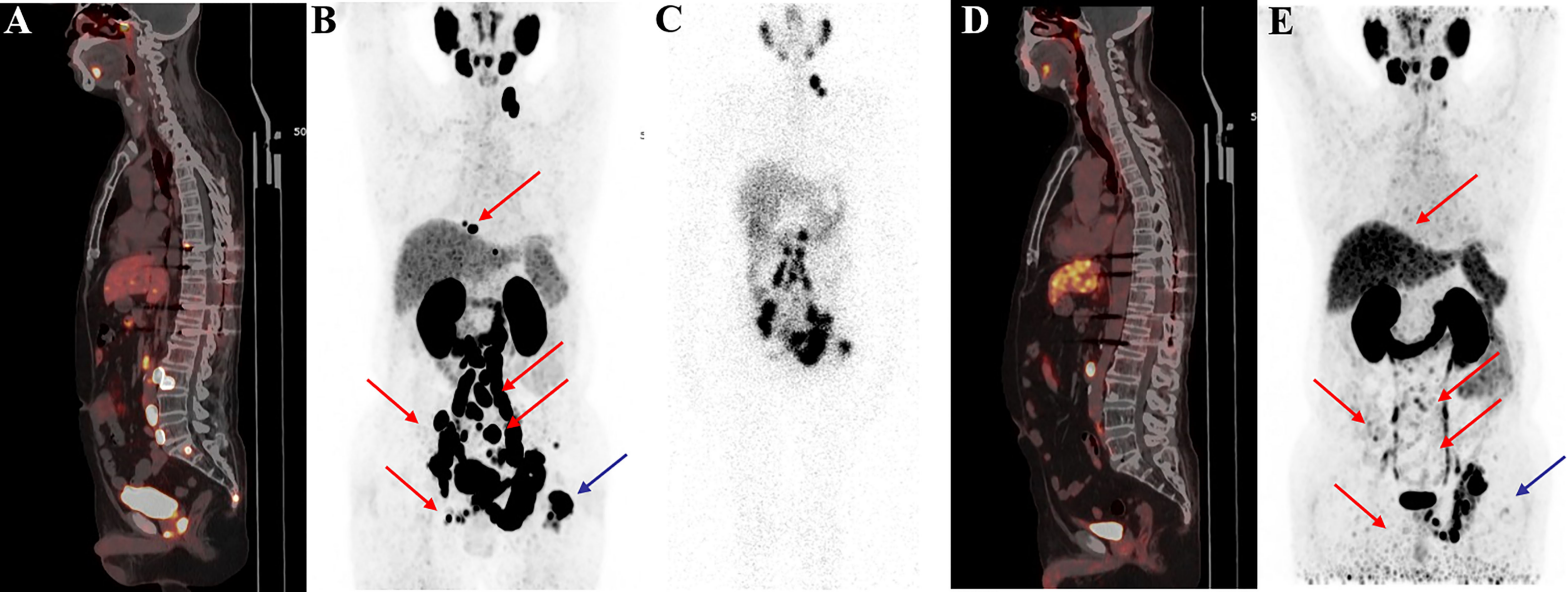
^68^Ga-PSMA-11 PET/CT in a 62-year-old patient with mCRPC. Baseline images (A, B) of multiple bone and lymph node metastases with increased PSMA expression. Post-therapy image (C) showing high ^177^Lu-PSMA-I&T aggregation in primary and metastatic lesions. Post-treatment image (D, E) showing multiple osteogenic metastases and reduced PSMA expression compared with before therapy. Metastatic foci in the left clavicle, abdominal aorta, and bilateral iliac paravascular lymph nodes were significantly reduced and PSMA expression was decreased, suggesting that the treatment was effective.
